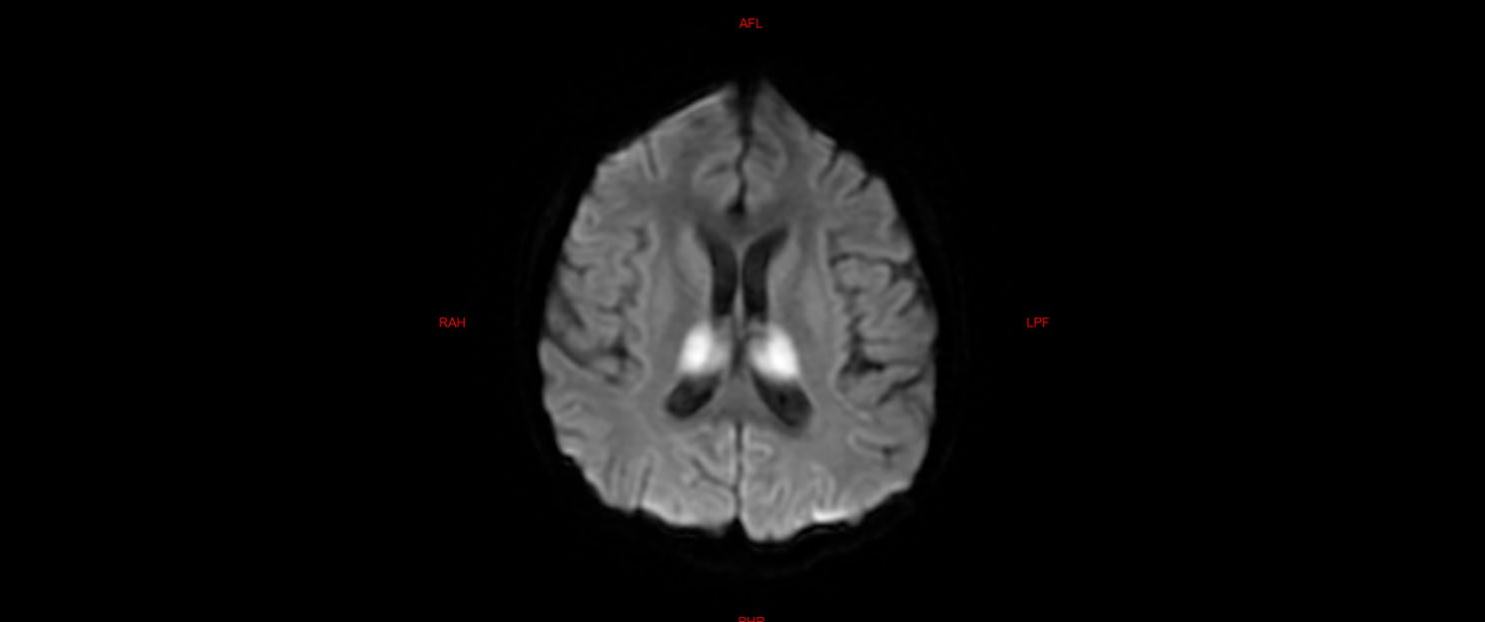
Brain MR DWI sequence of case 8, showing symmetrical diffuse high signal in bilateral thalamus. The patient, considered to have necrotizing encephalopathy, exhibited pronounced consciousness impairment upon admission, temporarily experiencing concomitant circulatory and respiratory failure. Following treatment, the patient was successfully weaned off advanced life support; however, there persisted severe cognitive regression and motor dysfunction
